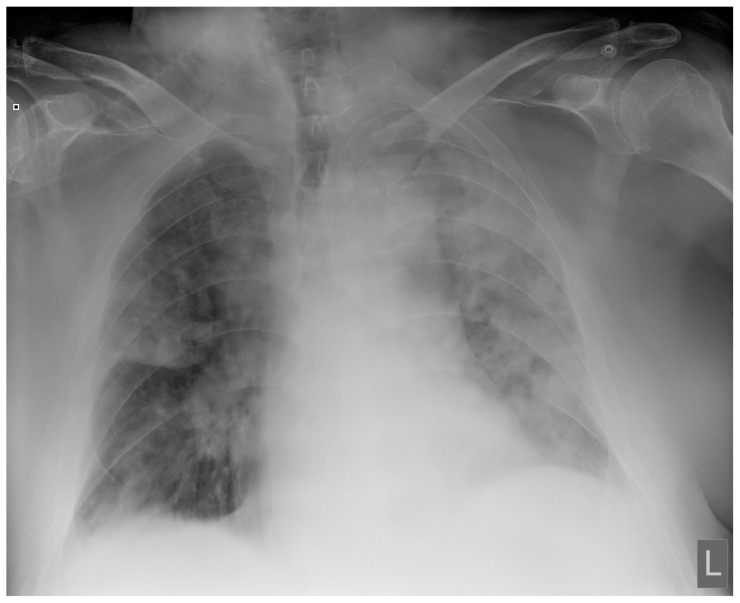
Chest X-ray upon admittance: patient 7. Bilateral inhomogenous infiltrates typical for COVID-19 pneumonia (the “L” orientation marker indicates the left side).
